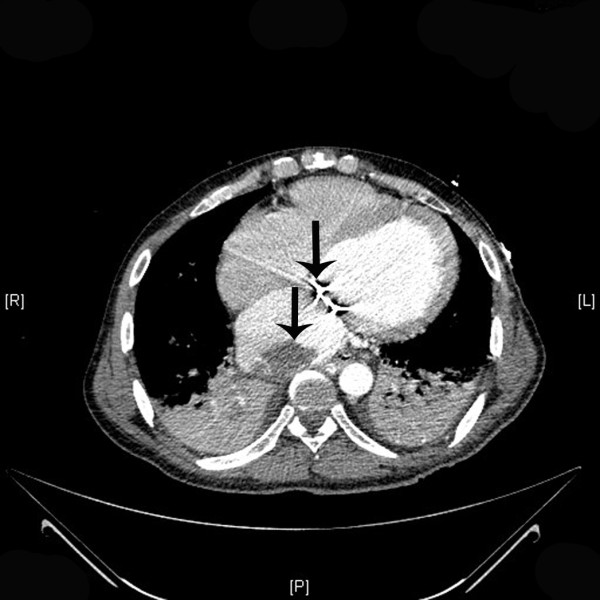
Transverse CT section at the heart level showing the artificial valve (upper arrow) and a thrombus in the left atrium (lower arrow).
